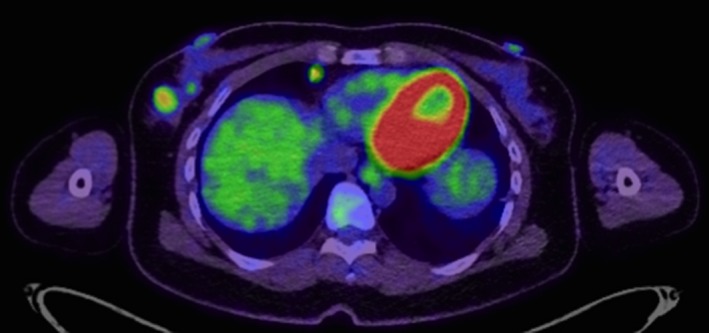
PET‐CT. A right breast mass with high FDG uptake (SUV max 4.97) is shown in the PET‐CT
